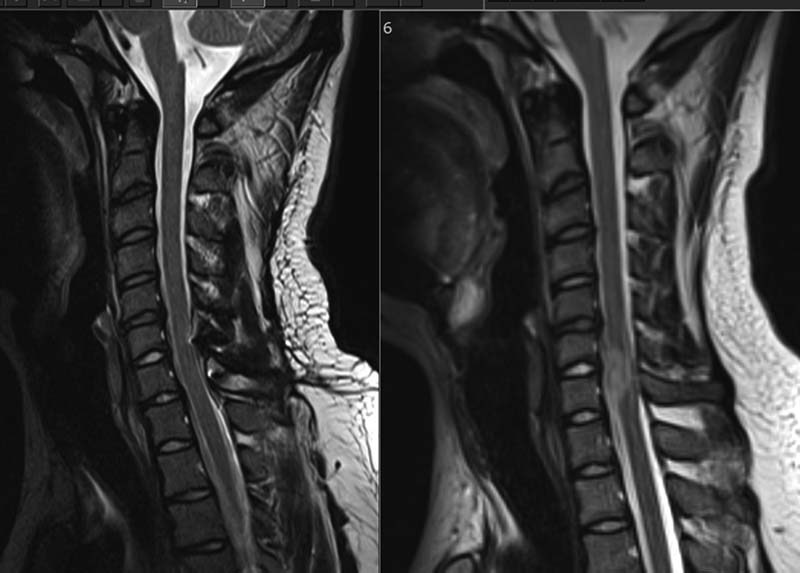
Sagittal T2-weighted magnetic resonance imaging of the first case showing intermediate signal of the lesion preoperatively (right) and postoperatively (left).
